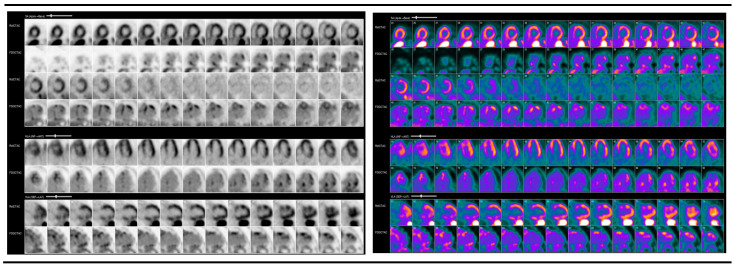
PET/CT demonstrating a “mismatch” pattern.
